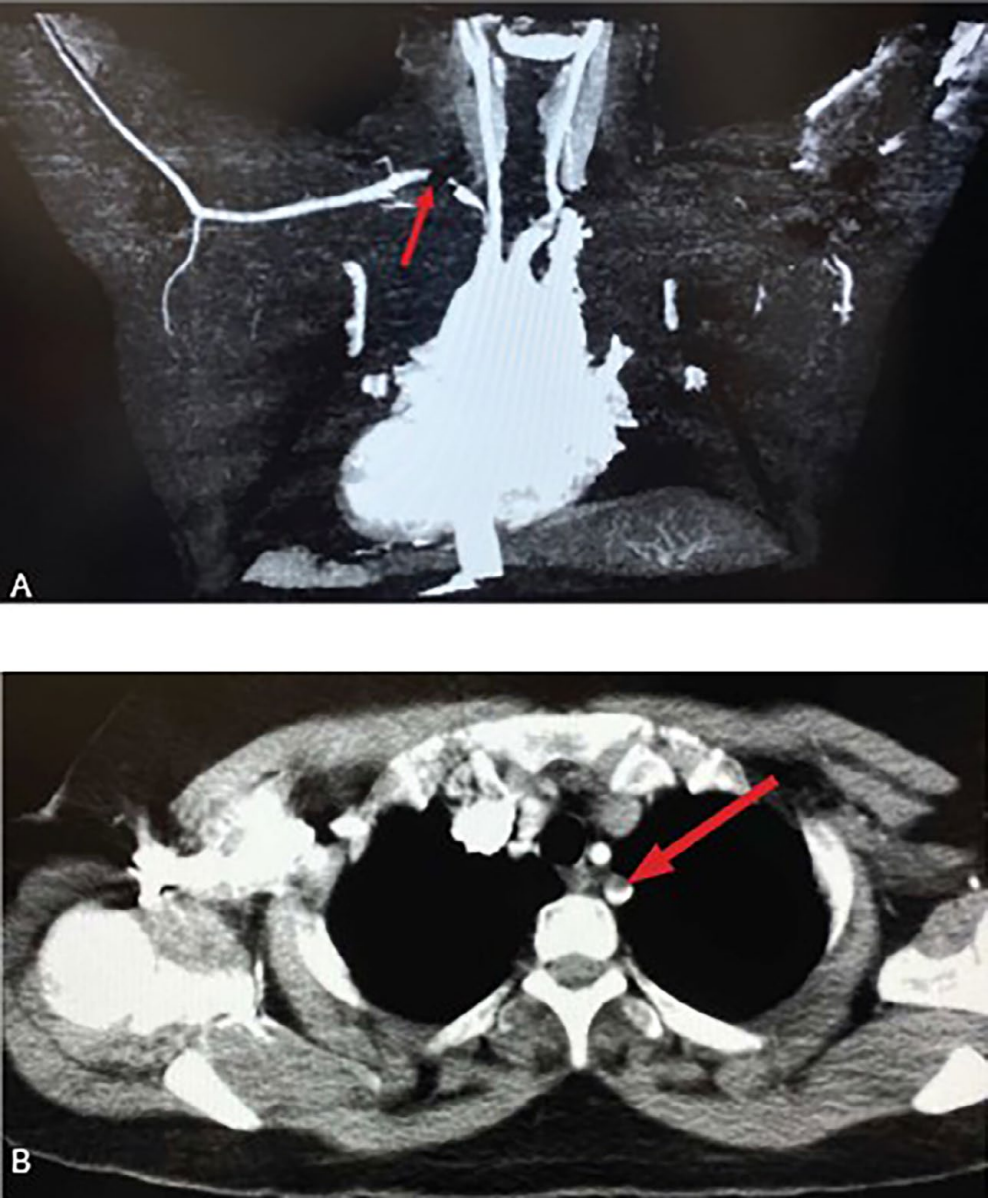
Displays two images obtained through CTA. In images A and B, the arrow points to the partial filling defects are observed in the proximal portion of the left subclavian artery.
